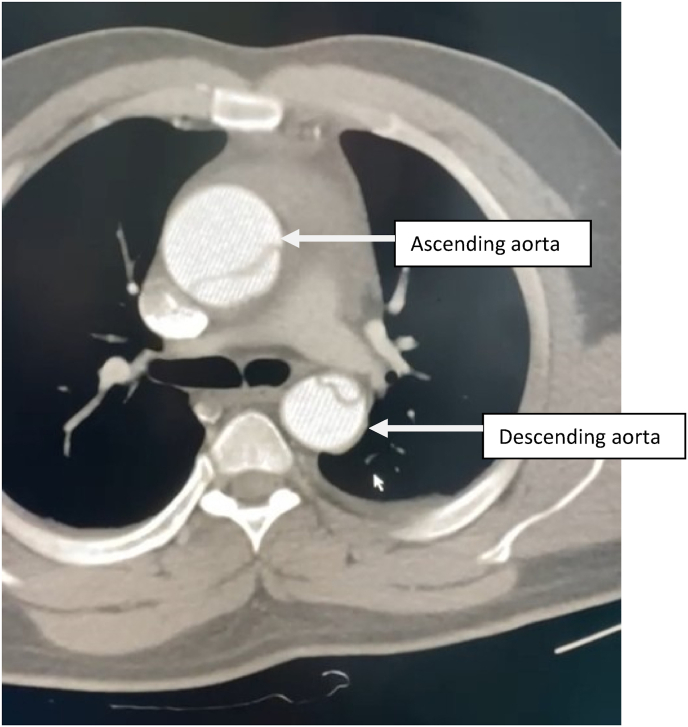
CT scan image showing the dissection in the ascending and the descending aorta.
